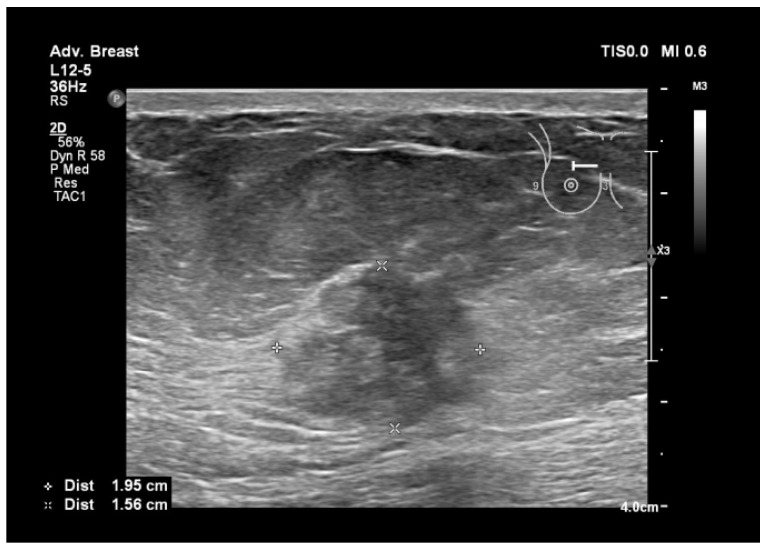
Breast lesion detected using ultrasound, shows a heterogenous, hypoechogenic solid nodule, with spiculations and ill-defined margins.
